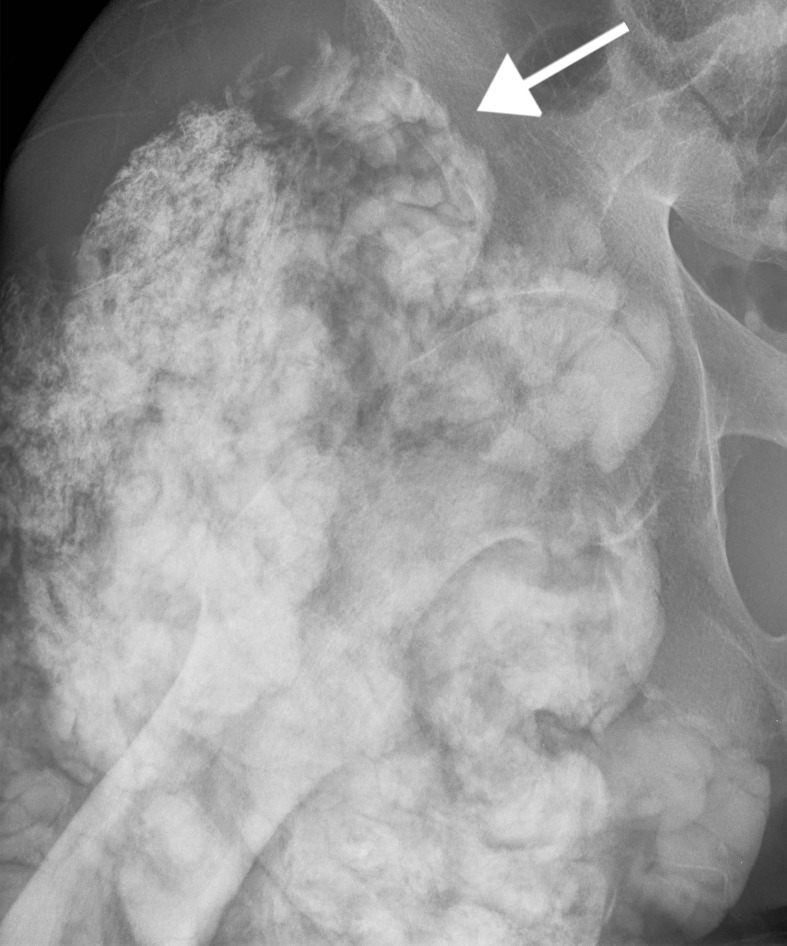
Right hip radiograph Anteroposterior (AP) radiograph of the right hip demonstrating large, dense, lobulated calcifications in a periarticular distribution (arrow).
